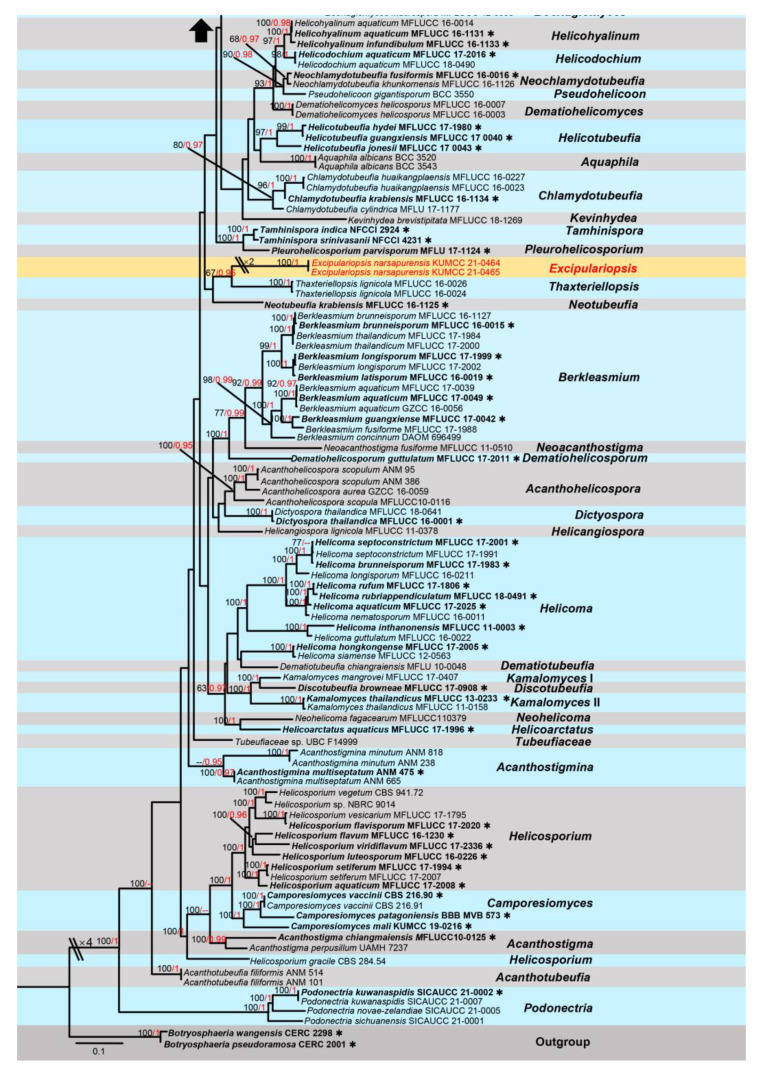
The phylogram based on a Maximum Likelihood analysis of combined LSU, *tef1*-*α*, *rpb2* and ITS sequence datasets. The analyzed gene contains 154 fungal strains and 3832 total characters including gaps (LSU: 1–853 bp, *tef1*-*α*: 854–1769 bp, *rpb2*: 1770–3228 bp, ITS: 2819–3438 bp). The tree topology of the ML resembles BI. The matrix had distinct alignment patterns, with the final ML optimization likelihood value of −59,582.416081 (ln). All free model parameters were estimated by RAxML model, with 1781 distinct alignment patterns and 21.90% of undetermined characters or gaps. Estimated base frequencies were as follows: A = 0.242692, C = 0.256407, G = 0.261351 and T = 0.239550, with substitution rates AC = 1.186006, AG = 5.169063, AT = 2.123954, CG = 0.808118, CT = 10.262422 and GT = 1.000000. The gamma distribution shape parameter alpha = 0.705889, and the Tree-Length = 10.099715. The final average standard deviation of split frequencies at the end of total MCMC generations was calculated as 0.009897 in BI analysis. The type strains are denoted in bold with the symbol “✱” at the ends, and newly introduced species in this study are denoted in red. The nodes provide bootstrap values of at least 60% (ML, left) and Bayesian posterior probabilities of at least 0.95 (BI, right); hyphens (-) signify values that are less than 60% in ML and less than 0.95 in BI. The bluish and pale brown backgrounds were used to distinguish different genera in Tubeufiaceae, while the yellow background indicates the genus *Excipulariopsis*.
